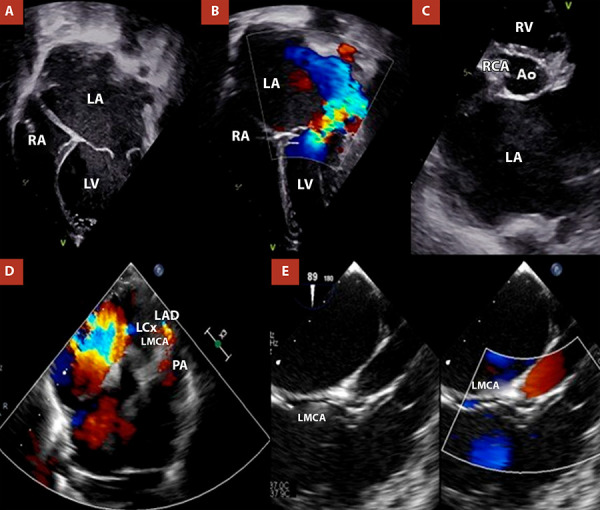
Transthoracic echocardiography (A, B) shows severe left atrial dilatation and severe mitral regurgitation. (C, D) The right coronary artery (RCA) is seen arising from the aorta (Z score +2.6), whereas the left main coronary artery (LMCA) originates from the pulmonary artery and bifurcates into the left circumflex artery (LCx) and the left anterior descending artery (LAD). Transoesophageal echocardiography (E), bicaval view, demonstrates the anomalous course of the LCT arising from the pulmonary artery.
